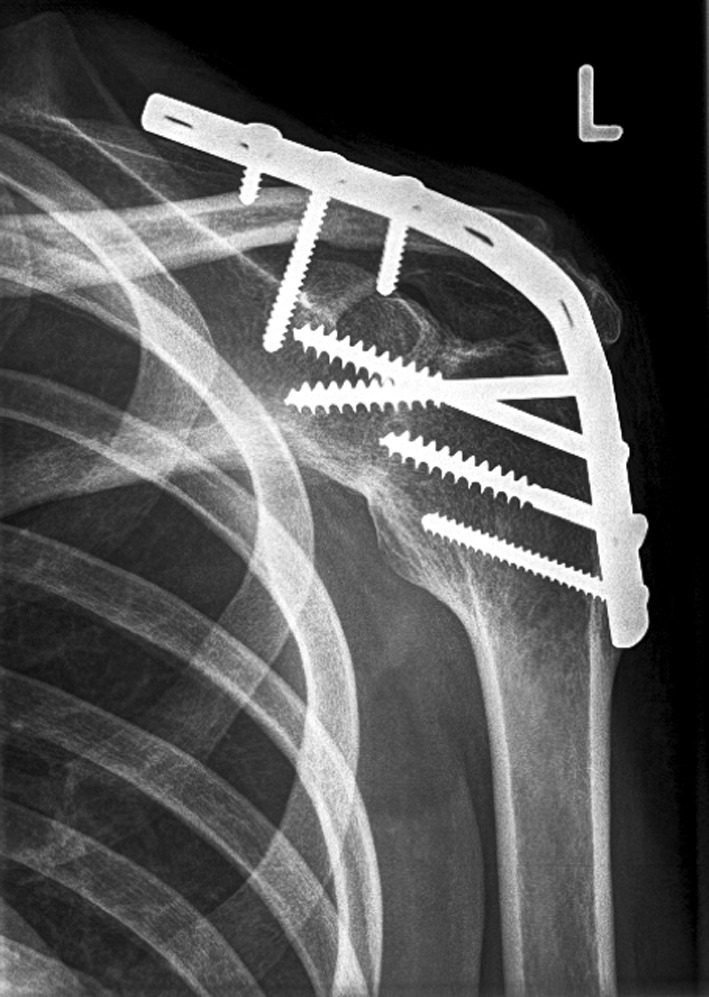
Anterior-posterior X-ray of the left shoulder of patient 12, 15.5-years after shoulder arthrodesis
